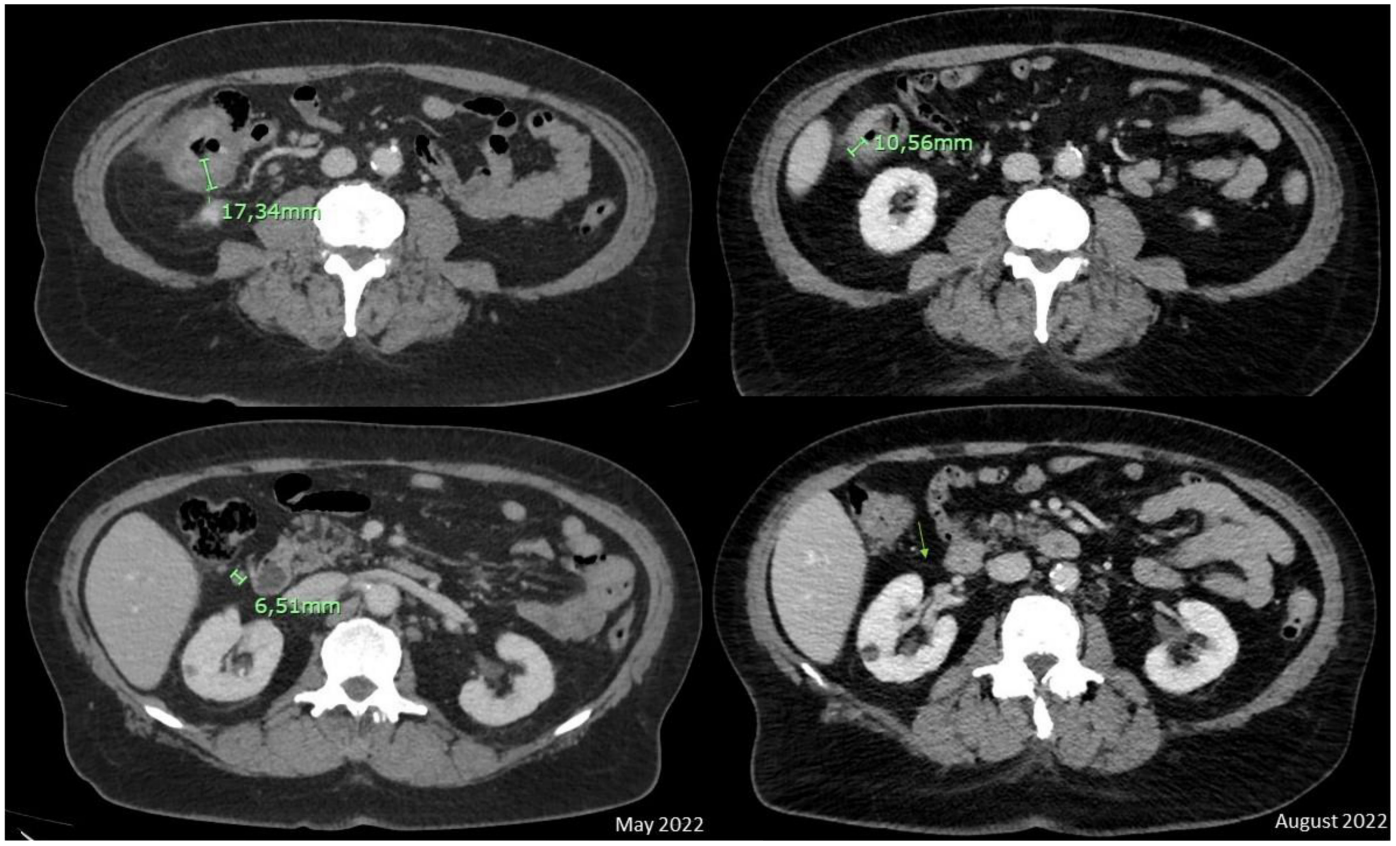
A major radiologic response was observed with a clear reduction of the colonic neoplasm and no longer evidence of adjacent suspicious nodes between the basal CT scan and interval CT scan in August 2022. The green arrow represents the absence of lymph node after 5 cycles of pembrolizumab.
